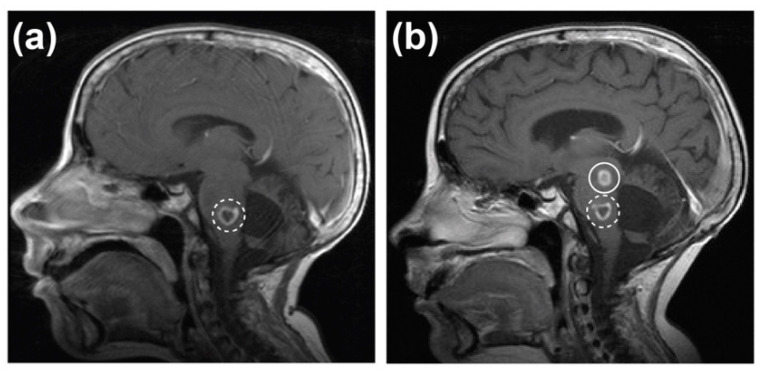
Magnetic Resonance Imaging (MRI) indicated midline glioma and fungal lesions within the brain. (a) MRI performed 11 years after initial medulloblastoma diagnosis depicting mass within the pons and upper medulla (dashed line). (b) MRI performed three months after initial diffuse intrinsic pontine glioma diagnosis depicting midline glioma (dashed line) and one of multiple suspected fungal lesions (solid line).
